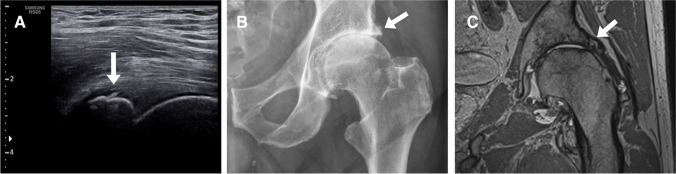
A 68-year old female with a large acetabular osteophyte (white arrow) visualized in longitudinal US (A), anteroposterior CR (B), and coronal PD-weighted MRI plane (C). The osteophyte is nicely delineated in each modality. Similarly as in the knee joint, the literature supports that US is at least comparable to CR for detection of hip joint osteophytes. Moreover, for the femoral head deformity, the diagnostic performance of the US was even superior to CR [68]
